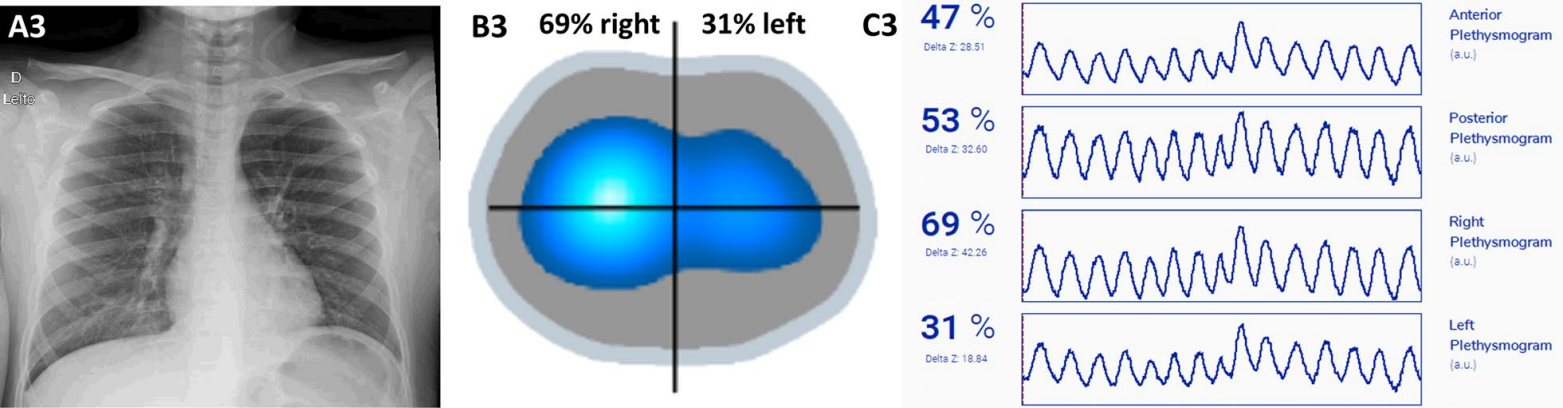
The figure shows chest radiograph images (A3), EIT images (B3) and plethysmogram (C3) of patient 3, a 10 years-old boy. The radiograph image shows elevation of the left diaphragm. EIT showed less variation in ventilation in the left region, compatible with the radiological image. The color scale of the EIT corresponds to the tidal volume variation present at the different ROIs; dark blue corresponds to a lower ventilation variation, and white corresponds to high tidal volumes. The plethysmogram (C3) shows the distribution of ventilation in the 4 ROis (upper, lower, right and left), and the amplitude of the curve indicates the variation in ventilation (delta Z), which is greater on the right lung (69%) than on the left lung (31%)
